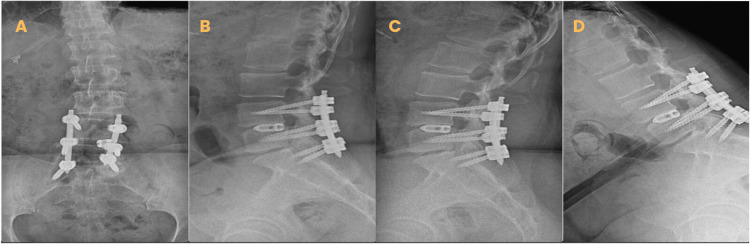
Radiography of lumbar spine. A, anteroposterior projection; B, lateral projection; C and D, dynamic projections. Images with posterior lumbar instrumentation L4-L5 + PLIF L4-L5
